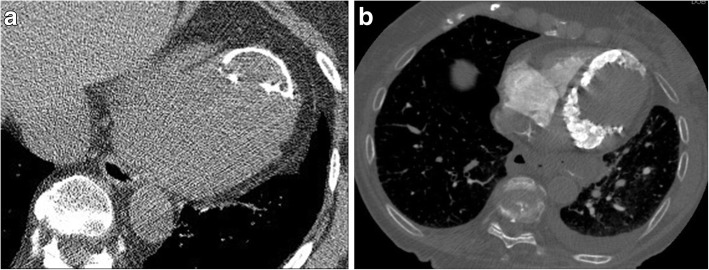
a, b Axial (a) and sagittal non-contrast CT image demonstrating curvilinear dystrophic calcification at the left ventricular apex in a 64-year-old female who was being evaluated for COPD. She had a history of previous myocardial infarct. b Axial non-contrast CT on bone window setting demonstrates metastatic myocardial calcification in the left ventricle in an 88-year-old woman with chronic renal impairment
